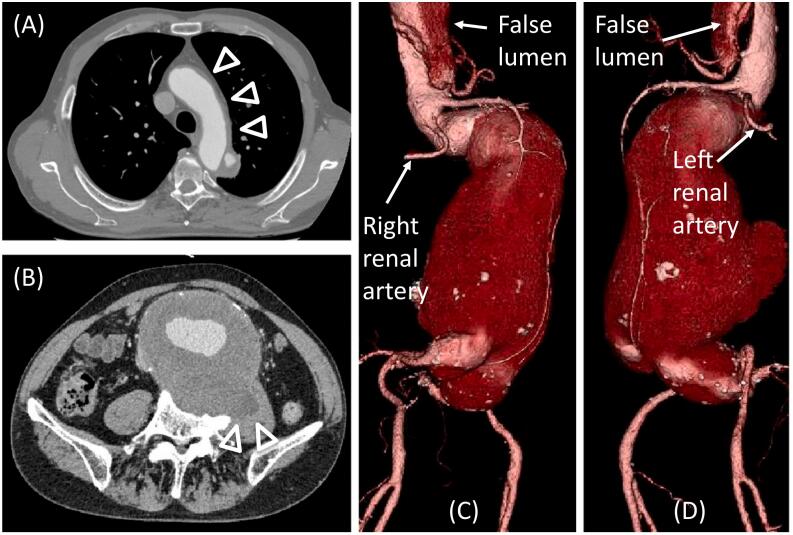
Preoperative enhanced computed tomography (CT) images. (A) False lumen from the ascending aorta to the aortic arch is occluded by thrombus (arrowheads). (B) Slight progression in the chronic rupture (arrowheads) compared to that at the time of initial admission is observed. Volume rendering images of the CT angiography on the right anterior oblique position (C) and left anterior oblique position (D) show almost no enlargement in the size of the AAA. The false lumen was patent at the vicinity of the right renal artery.
